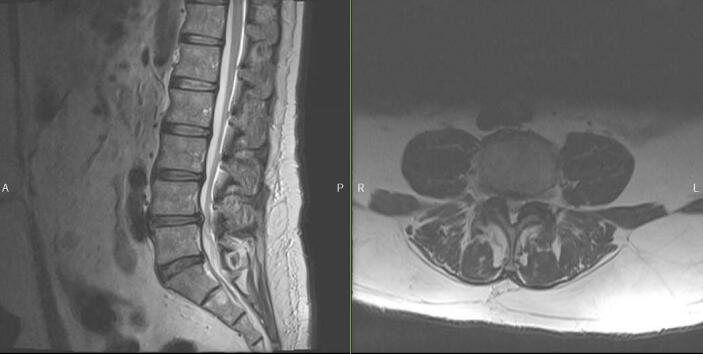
Sagittal and axial MRI images demonstrating severe L4-5 central stenosis from disc protrusion, ligamentum flavum hypertrophy, and facet hypertrophy.
